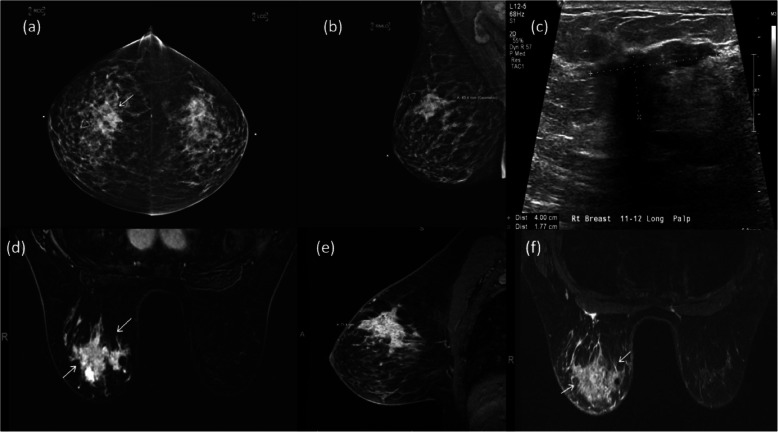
A 59-y/o female presented with palpable mass of right upper breast. Biopsy revealed intraductal carcinoma (IDC) grade III. (a) Digital mammography cranial-caudal (CC) views of both breasts. Notice the lesion (arrows) was poorly delineated due to dense breast. (b) Mediolateral oblique (MLO) view indicates longest dimension of lesion to be 4.3 cm. (c) Under targeted US, the lesion was seen as ill-defined hypoechoic mass with longest dimension of 4 cm. Axial and sagittal post-contrast, fat-suppressed dynamic contrast enhanced (DCE) MRI shows the lesion of a much larger extension at 7.3 cm and avidly enhancing (d and e, arrows). (f) Axial fat-suppressed T2-weighted image shows the large carcinoma (arrows) as hyperintense lesion involving most of the upper part of the breast. Images courtesy of Maia Rauch, MD, PhD
